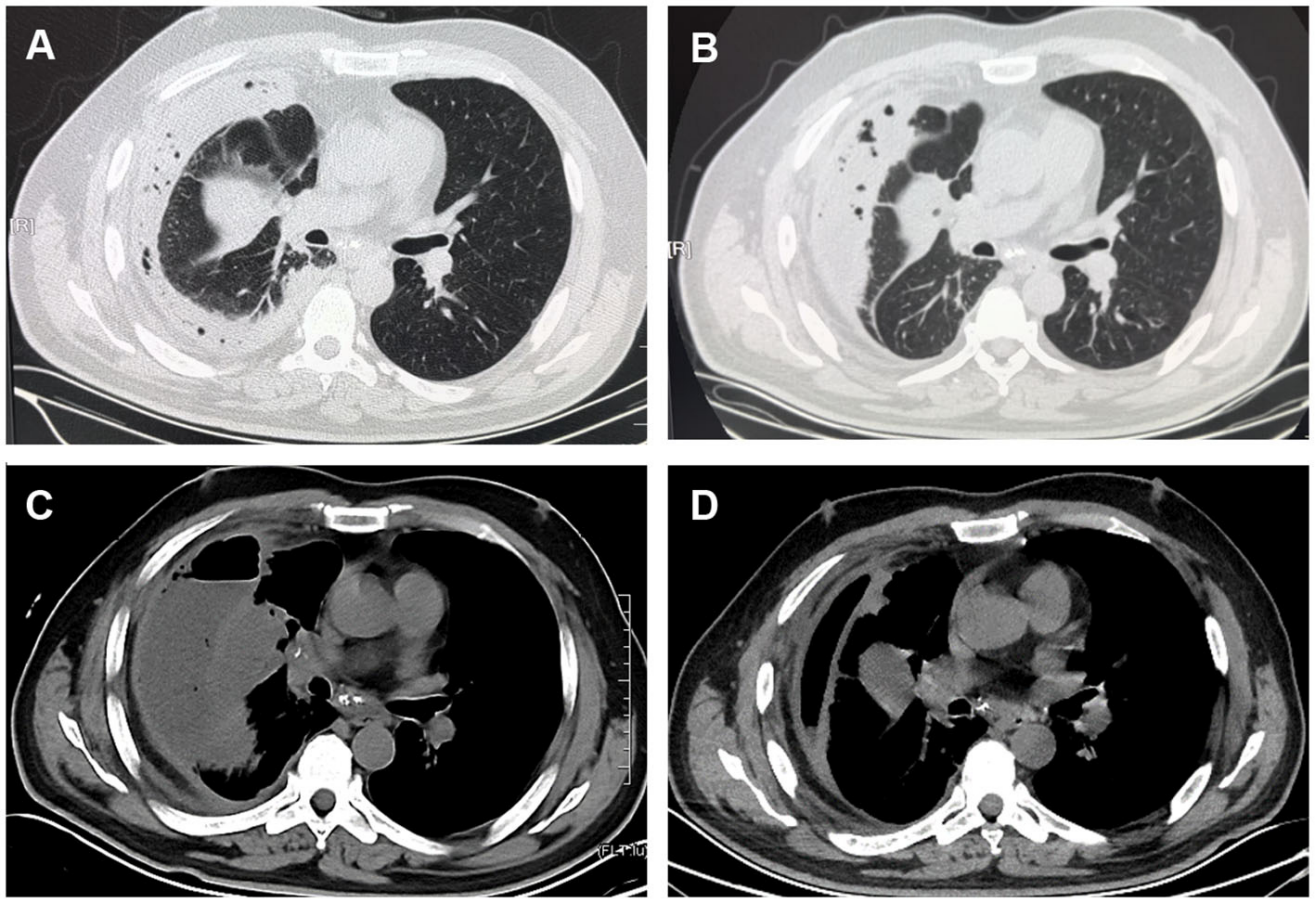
CT scan images. (A) Chest CT showed an irregular mass at the right middle lobe of the lung near the interlobar fissure with right atelectasis and encapsulated effusion and pneumatosis. (B) Chest CT showed no significant changes in the amount of right-sided pleural effusion after thorough pleural drainage and anti-infective treatment for 3 weeks. (C) Contrast-enhanced CT scan showed uneven enhancement of the mass in the lateral segment of right middle lobe, mediastinal lymphadenopathy with visible enhancement, and a right hydropneumothorax on April 1. (D) Two weeks after readmission, chest CT showed that the right-sided pleural effusion and gas were significantly reduced.
